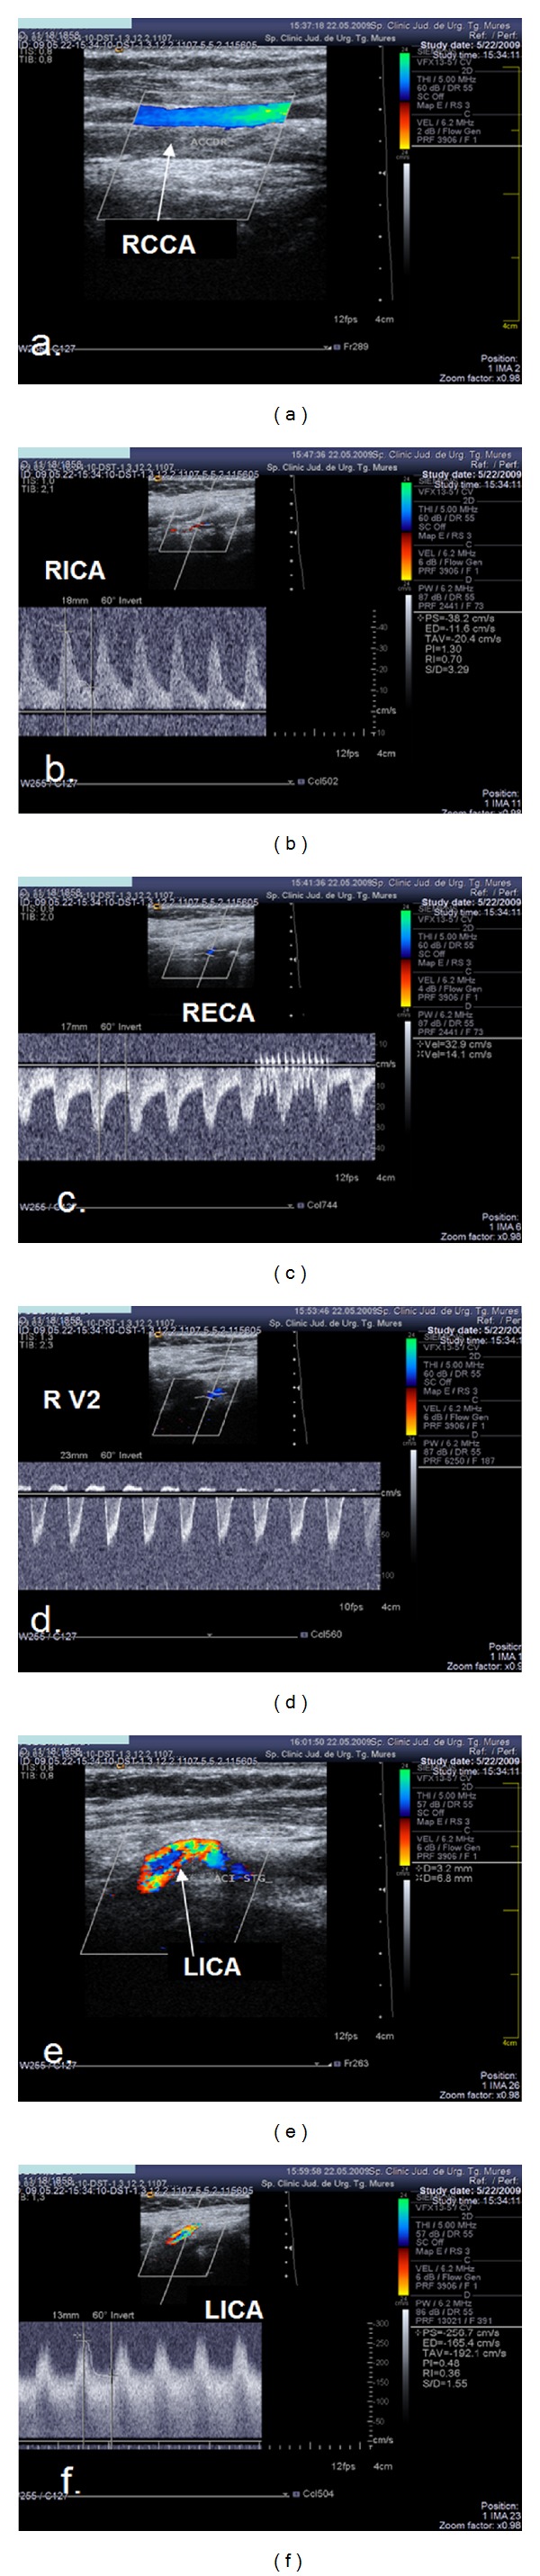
Ultrasound examination showing multiple steno-occlusive lesions in the cervical vessels. (a) Colour mode examination: RCCA occlusion, no flow in the right common carotid artery, and the vessel lumen is filled with thrombotic material. (b), (c) Duplex mode examination: anterograde flow in the right ICA and retrograde flow in the right ECA. (d) Duplex mode examination, subclavian steal phenomenon, and retrograde flow in the right vertebral artery. (e), (f) Colour mode and duplex mode examination and severe left internal carotid artery stenosis.
